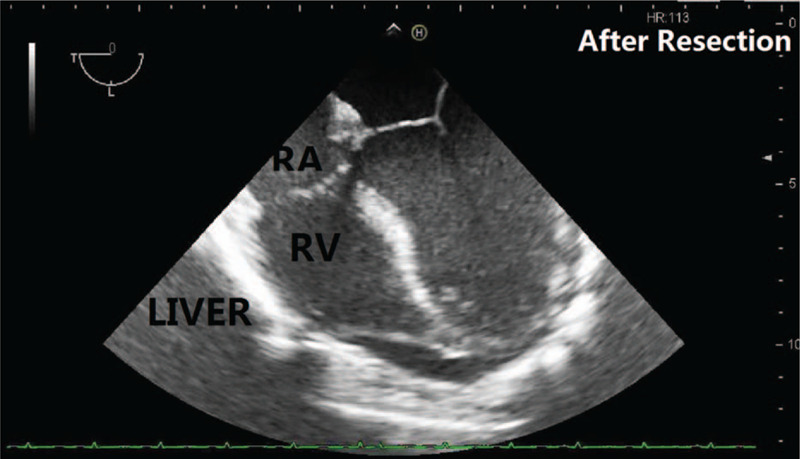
TEE image (ME 4C) of the first patient after the resection. The patient's heart returned to its normal anatomic position, and the right atrium was well filled. TEE = transesophageal echocardiography.
